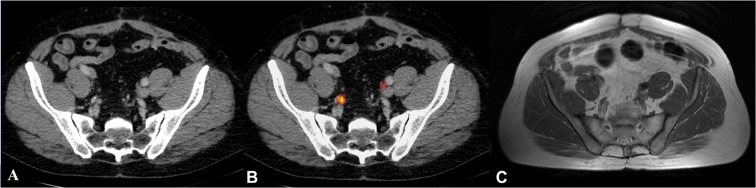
Example of a lymph node which is challenging to detect on T1 MRI compared to the ^68^Gallium-Prostate specific membrane antigen PET-CT. This figure presents an example of a lymph node close to the iliac bifurcation. The right and left external and internal iliac bifurcations are visualized in corresponding axial plane slices using CT, ^68^Gallium-Prostate specific membrane antigen PET-CT and T1 sequence MRI presented left to right. (A) Lymph node depicted in CT, (B) Lymph node depicted in ^68^Gallium-Prostate specific membrane antigen PET-CT; (C) Lymph node depicted in the T1 sequence of MRI.
